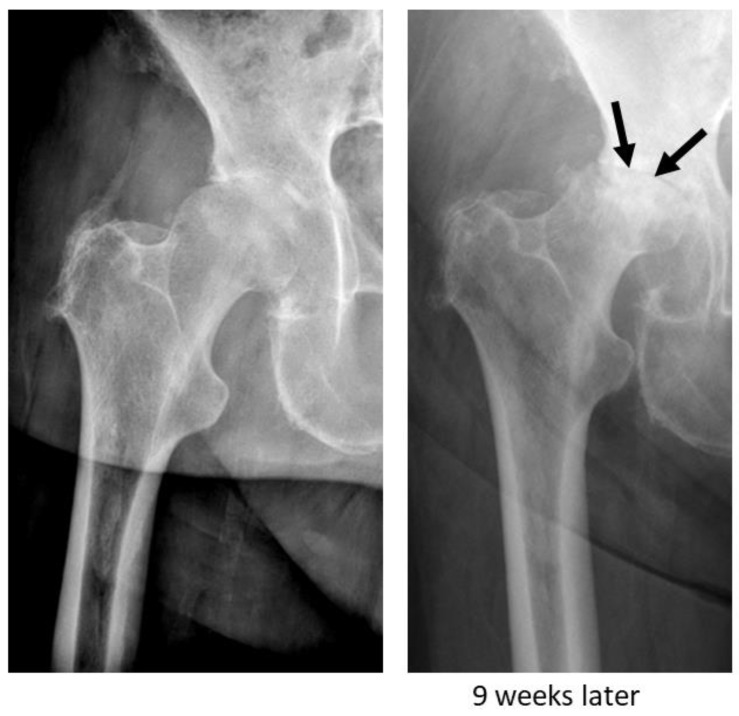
X-ray of a right hip joint (ap) of a 77-year-old female patient with osteoarthritis (left) and X-ray 9 weeks later showing rapidly destructive osteoarthritis (arrow) (right). The patient had no known risk factors for osteoporosis.
